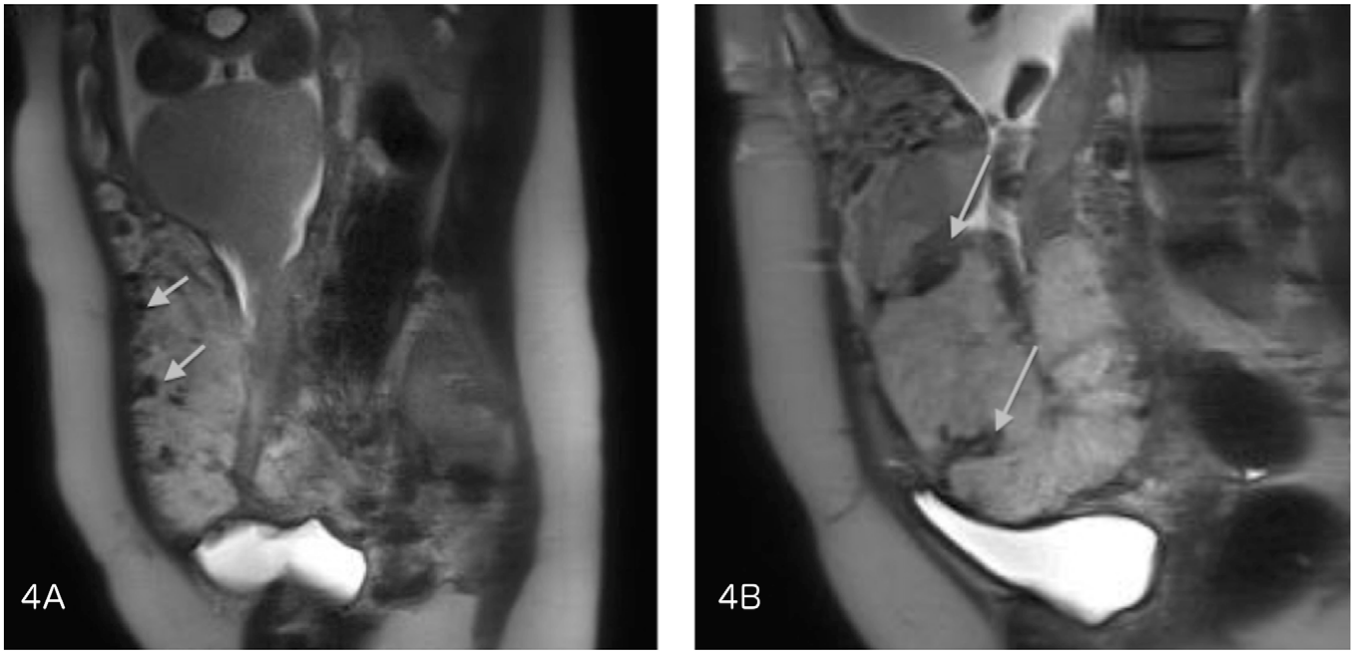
A–B - Dark intraplacental bands on T2-weighted images on MRI.
